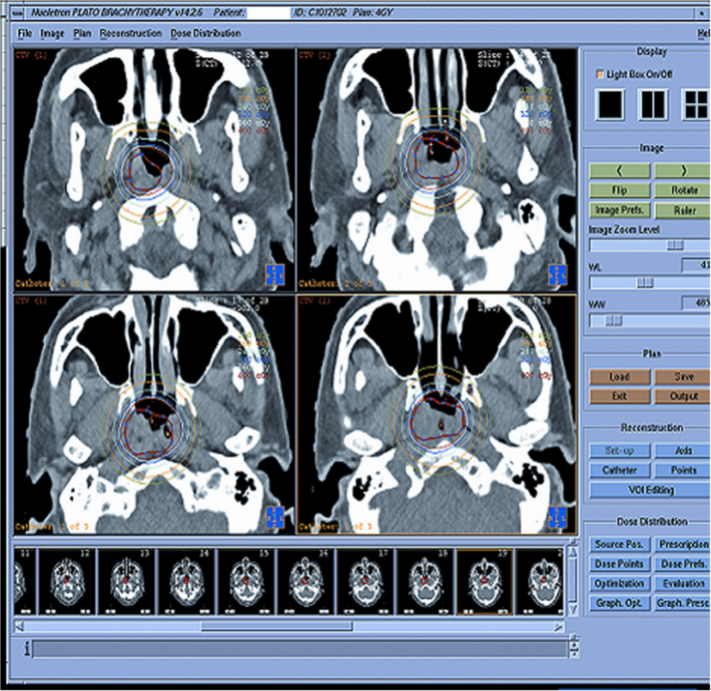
Axial computed tomography (CT) image showed the applicators *in situ *for brachytherapy intracavitary with different isodose lines.
